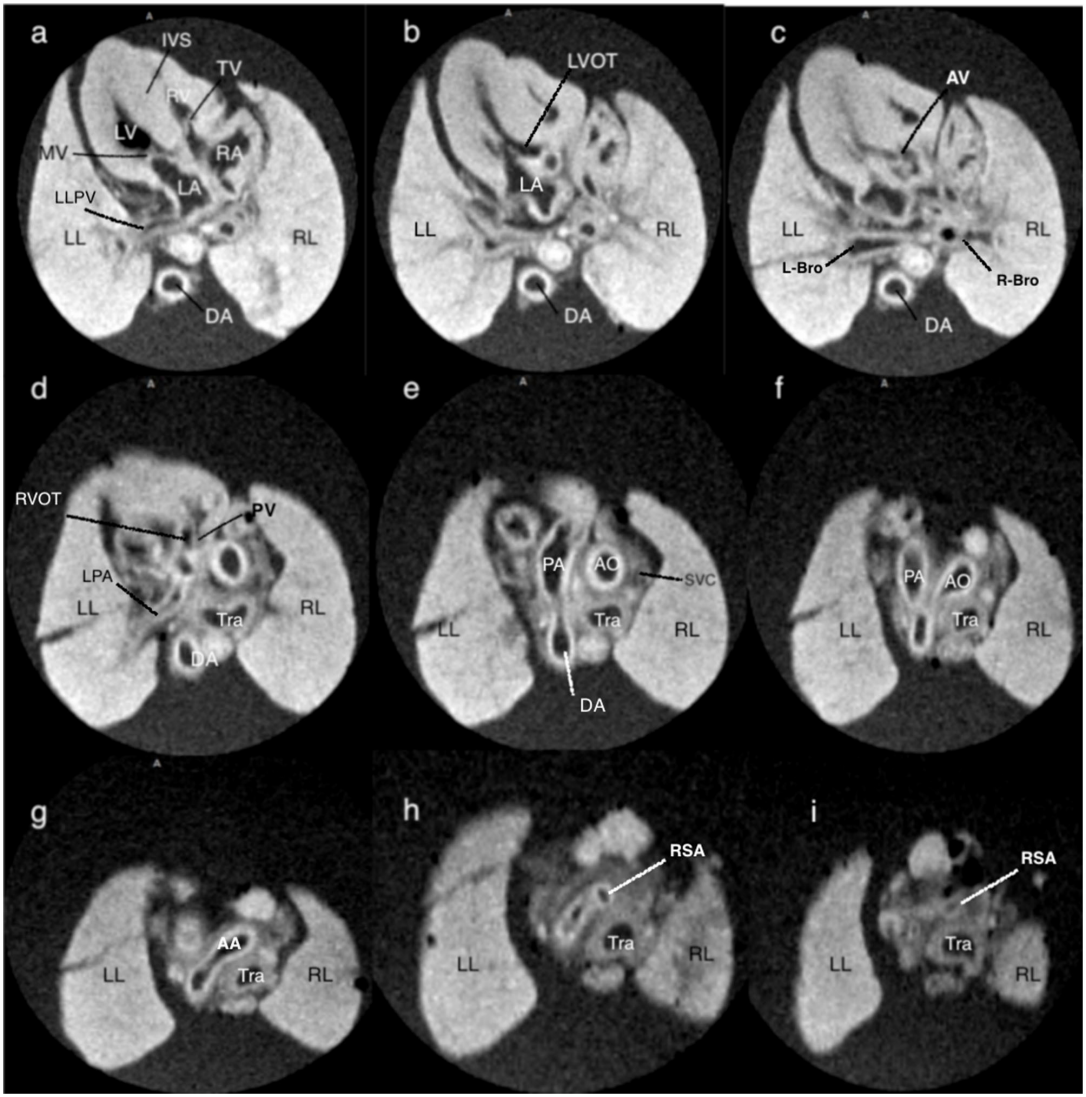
T1-weighted magnetic resonance consecutive images at 9.4 T of a fetus with normal cardiac anatomy, following termination of pregnancy at 13+1 weeks of gestation. (a) at the level of four-chamber view, showing a normal situs of the heart surrounded by the lungs, and a normal four-chamber view. (b,c) at the level of the left outflow tract showing the medial aortic wall was continuous with the ventricular septum and aortic valve. (d) at the level of the right outflow tract with a pulmonary artery that originates from the right ventricle with the clear bifurcation of the main pulmonary artery and left pulmonary artery. (e) at the level of the three-vessel view, the main pulmonary artery, ascending aorta, and superior vena cava are arranged in a straight line that extends from the left anterior to the right posterior. (f) at the level of the three-vessel and trachea view showing the transverse aortic arch and isthmus merge into the descending aorta, as does the pulmonary trunk and ductus arteriosus, creating a V-shaped configuration. (g–i) showing the right subclavian artery that branches off the aorta. LA, left atrium; LV, left ventricle; RA, right atrium; RV, right ventricle; RL, right lung; LL, left lung; TV, tricuspid valve; MV, mitral valve; LLPA, left lung pulmonary vein; DA, descending thoracic aorta; IVS, interventricular septum; LVOT, left ventricle outflow tract; AV, aortic valve; L-Bro, left bronchus; R-Bro, right bronchus; LPA, left pulmonary vein; RVOT, right ventricle outflow tract; Tra, trachea; PA, pulmonary artery; AO, aorta; AA, aortic arch; SVC, superior vena cava; RSA, right subclavian artery.
